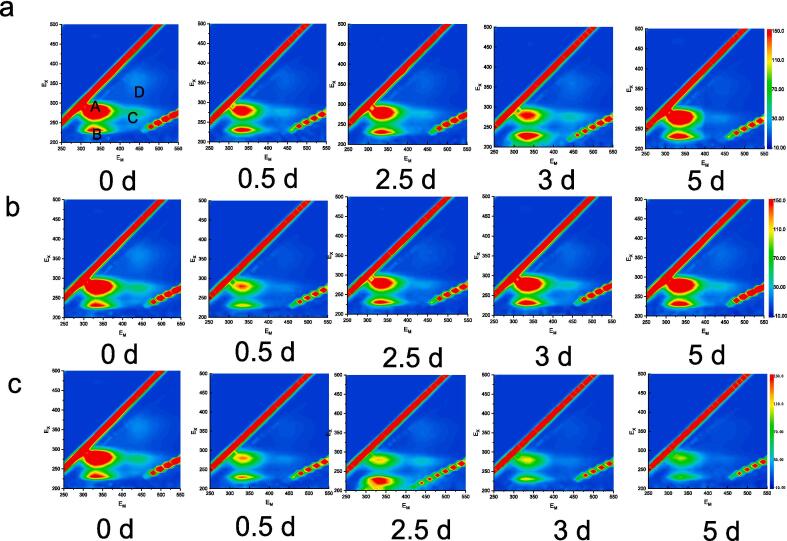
Excitation-Emission Matrix (EEM) of Intracellular Organic Matter (IOM) Changes. (a) Control; (b) Single ultrasound treatment; (c) Second ultrasound treatment. The single ultrasound treatment consisted of a 20-minute session on the first day. The second ultrasound treatment involved a subsequent 20-minute session on the 2.5th day, following the initial treatment.
